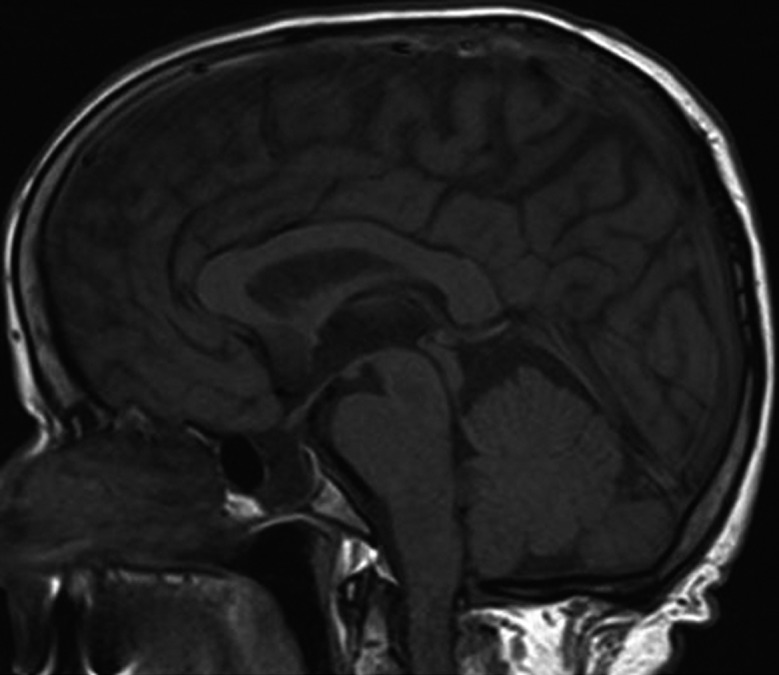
Cerebral MRI, T1-weighted, sagittal midline. Platybasia, short clivus, and small posterior cranial fossa. Arachnoidocele of the sella turcica.
